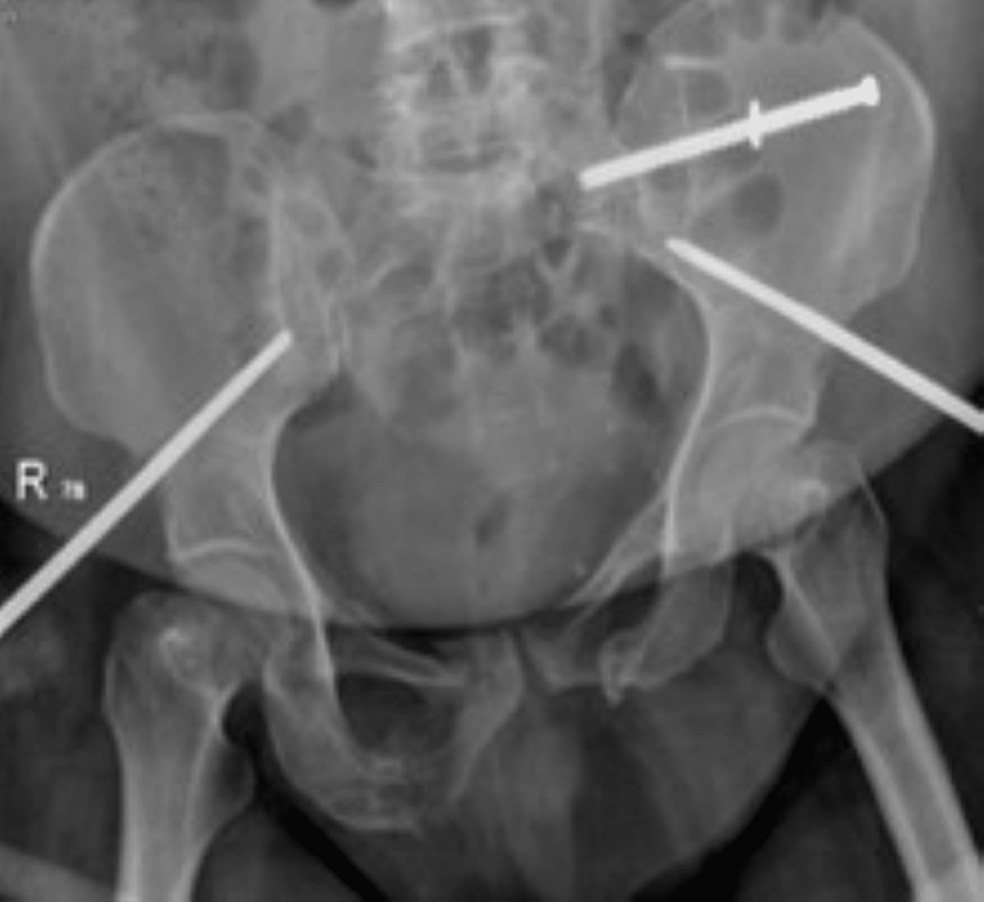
Radiograph demonstrating a pulled-out sacroiliac screw with a stable fracture on follow-up.
